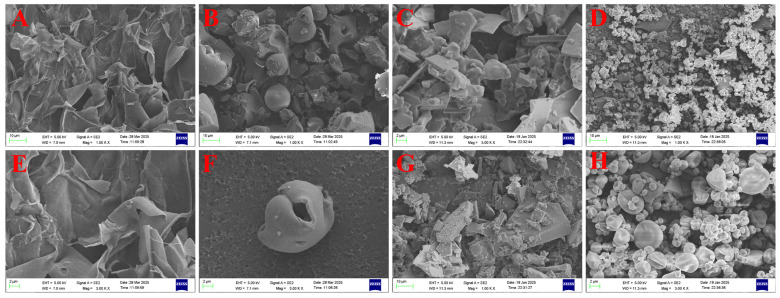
SEM images of CPE, CT powder, FDMCs, and SDMCs. (A): CPE (1K×); (B): CT powder (1K×); (C): FDMCs (1K×); (D): SDMCs (1K×); (E): CPE (3K×); (F): CT powder (3K×); (G): FDMCs (3K×); (H): SDMCs (3K×). CT: corn peptide; CPE: Chenpi extract; FDMCs: freeze-dried microcapsules; SDMCs: spray-dried microcapsules.
